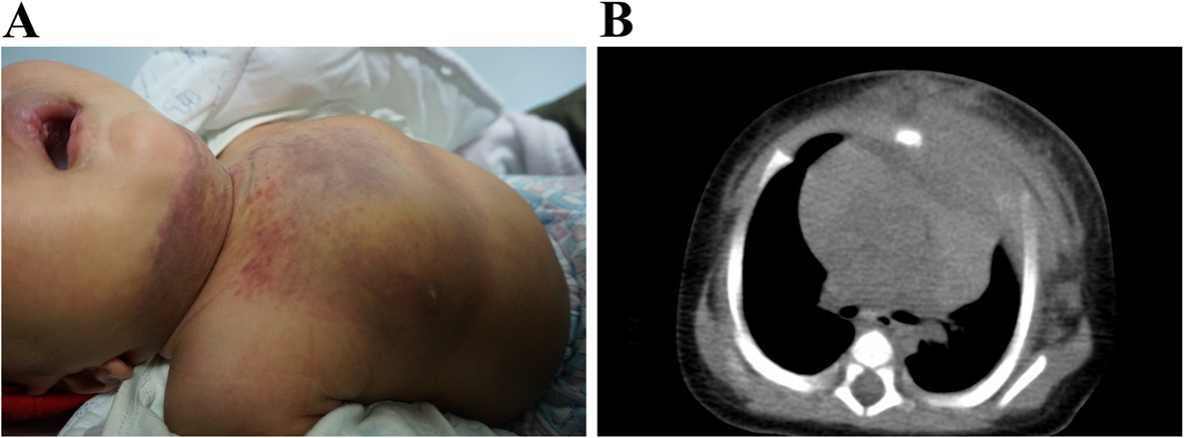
KHE with cutaneous involvement. a The photograph shows an indurated and purpuric mass in the anterior neck, chest and abdominal wall (Patient #13). Ecchymosis was evident with telangiectasia. b An unenhanced CT scan revealed an extensive soft-tissue mass with ill-defined margins throughout the anterior thoracic musculature
